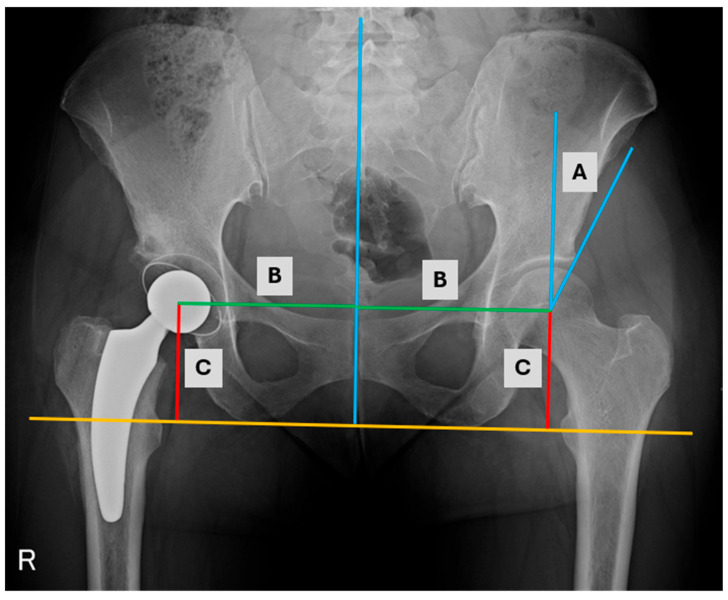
AP radiograph of the hips with measurements (A) Wiberg angle, (B) medialization, (C) distalization.
